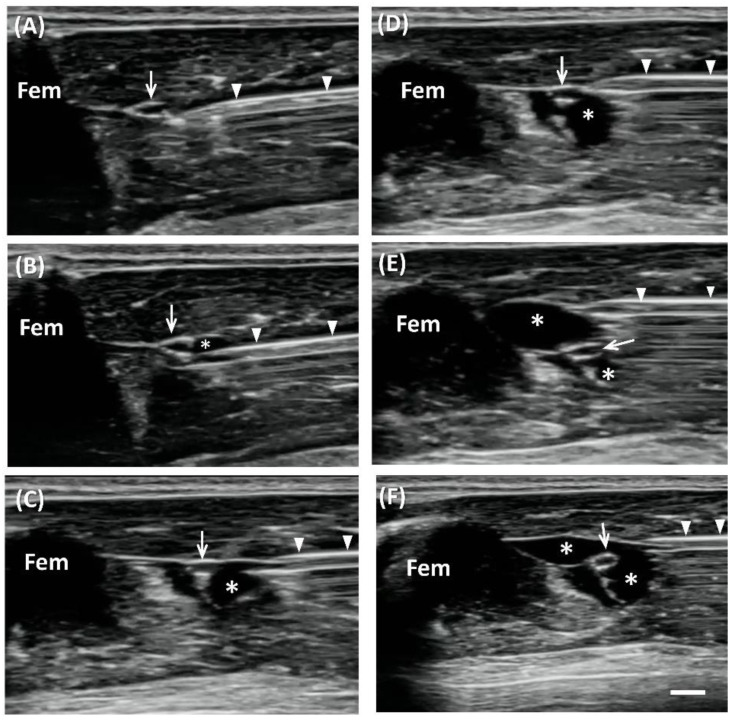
Ultrasound (US)-guided perineural injection of hydrogel. Short-axis view, in-plane approach of US-guided perineural injection of hydrogel (asterisks) showing the insertion of the needle (arrowheads) in the deep regions around the sciatic nerve (arrow) initially (A–C), and then superficially (D–F) (Fem, femur) (Scale bar: 1 mm).
